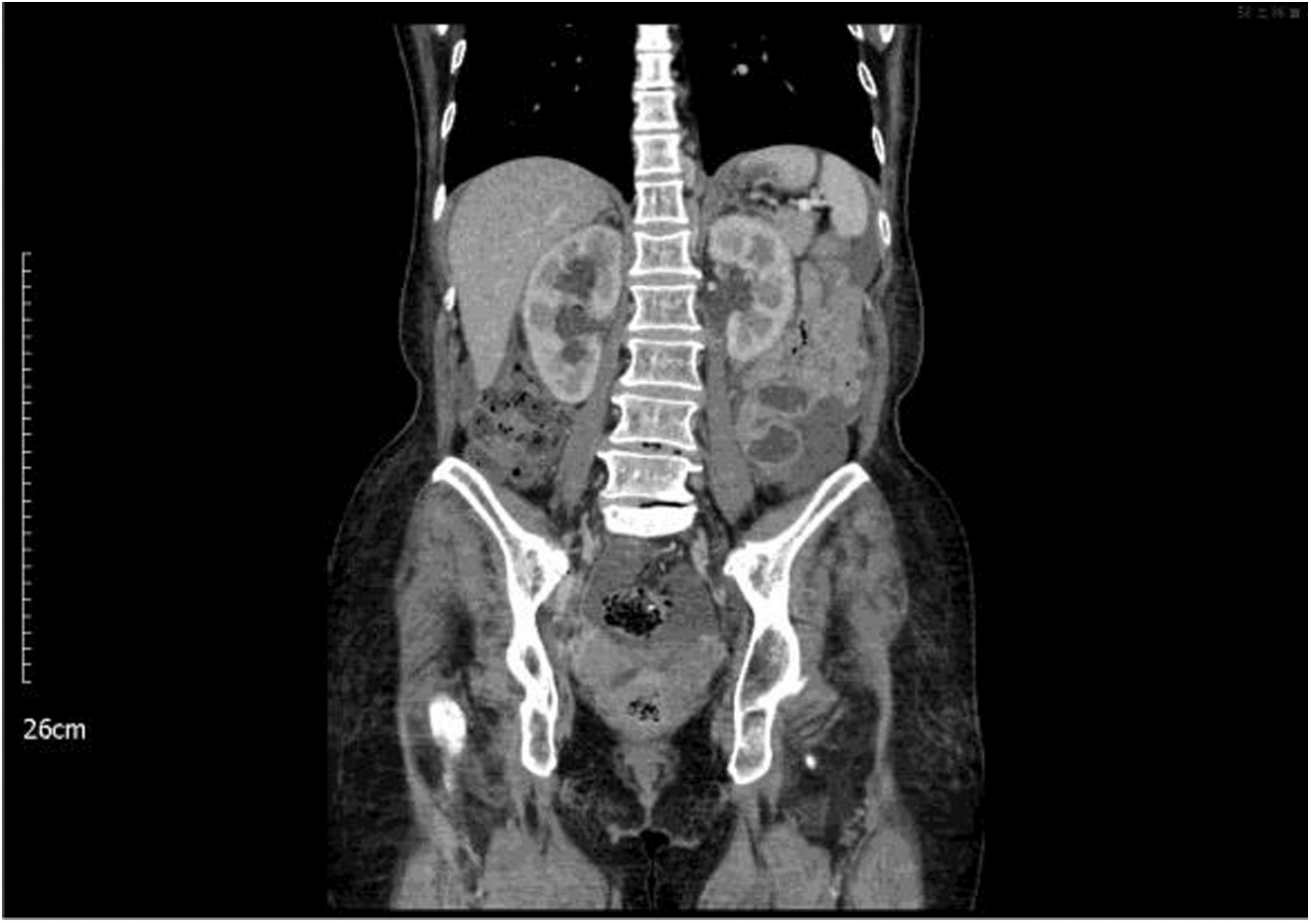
Abdominal computed tomography showing strictures in the lower third of bilateral ureters with bilateral hydronephrosis.
